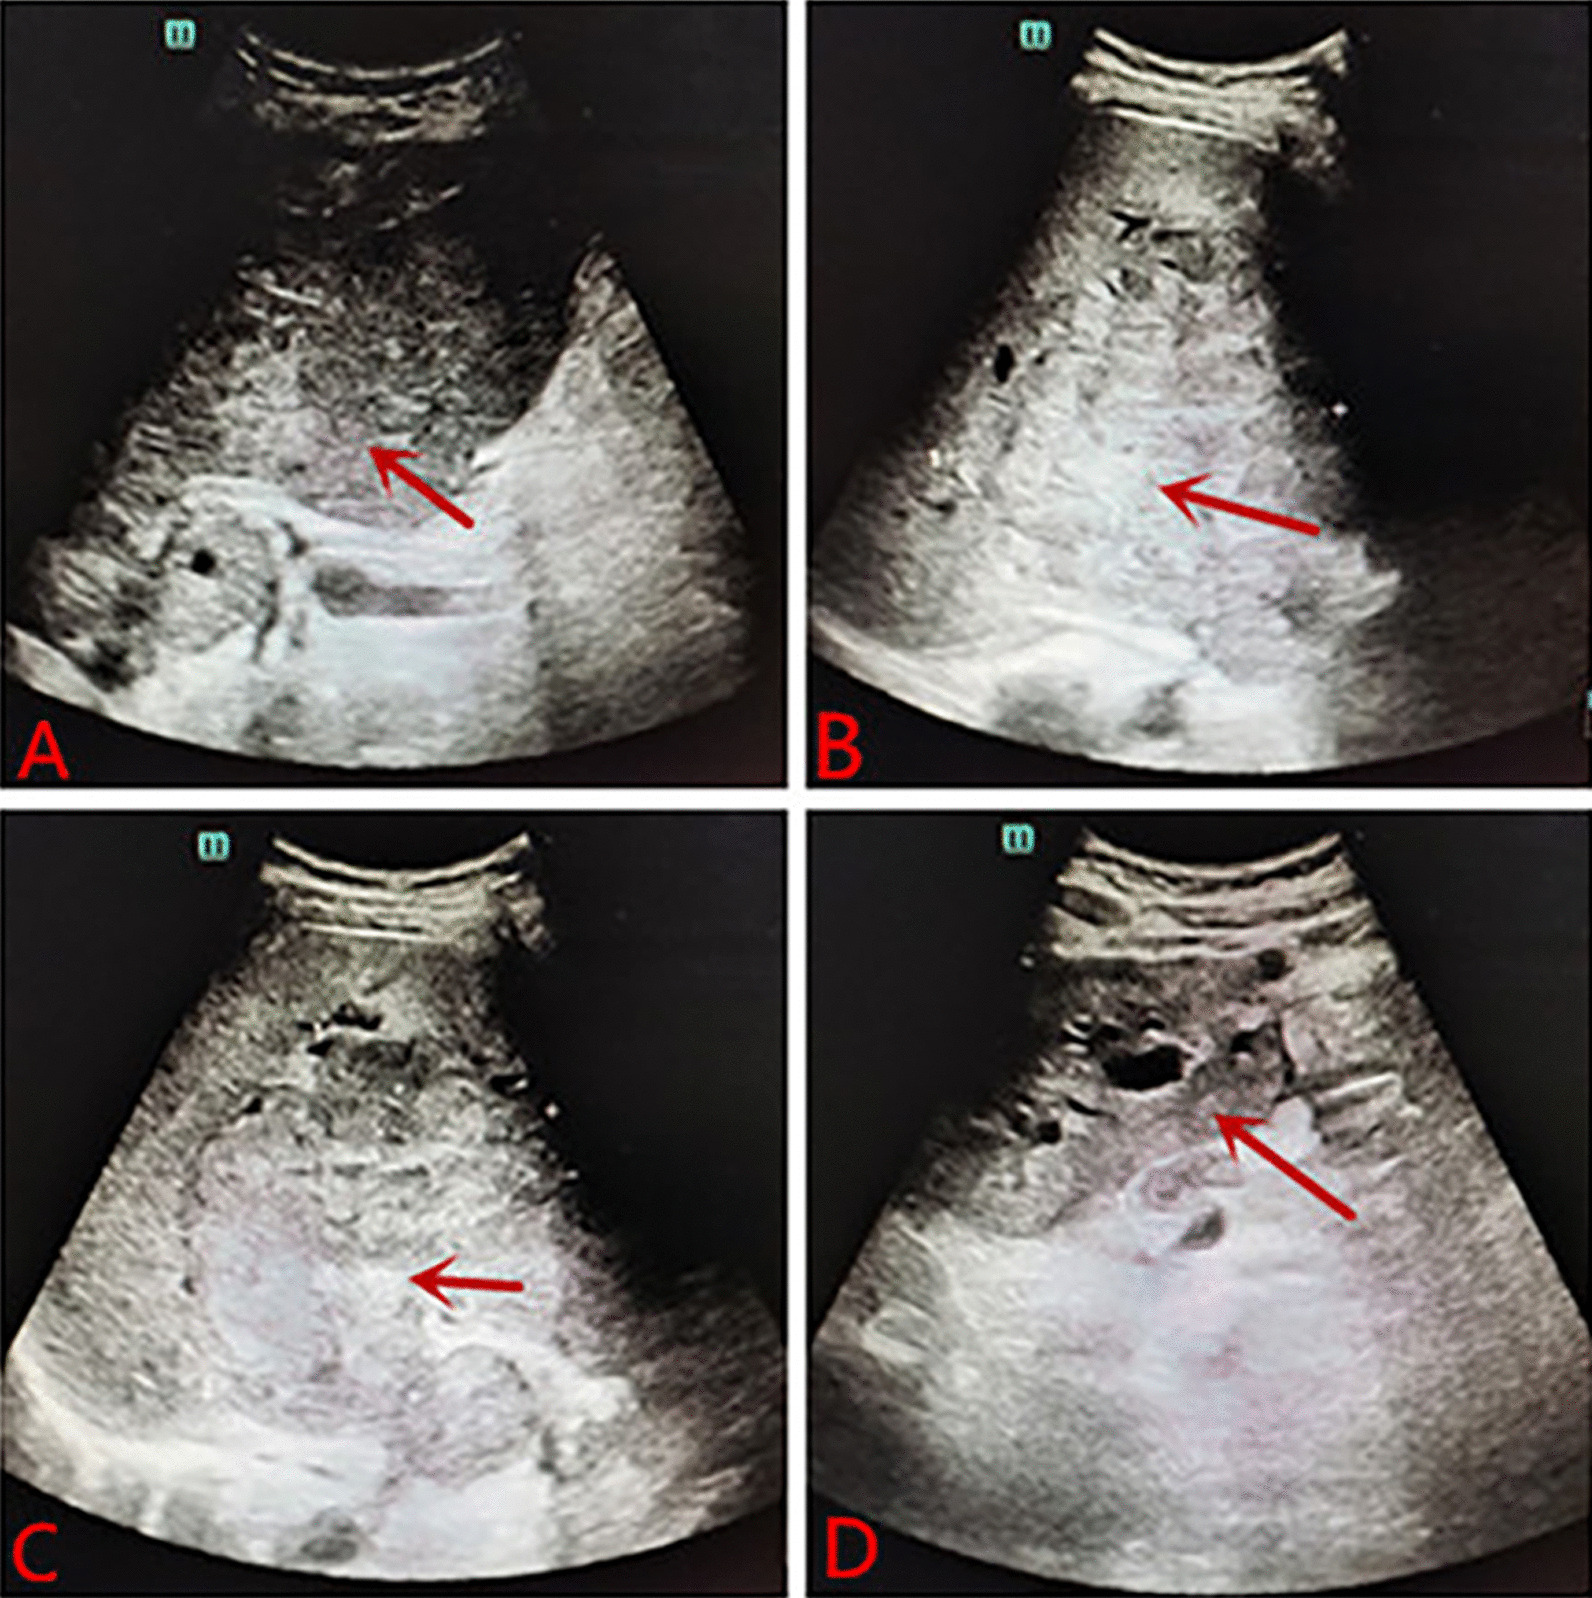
A Abdominal ultrasound: size increased liver, and multiple hypoechoic masses observed. B–D Multiple hypoechoic masses in liver, the large intrahepatic mass is about 11.5 * 10.8 * 10.2 cm, located at the right, consider the possibility of liver cancer
